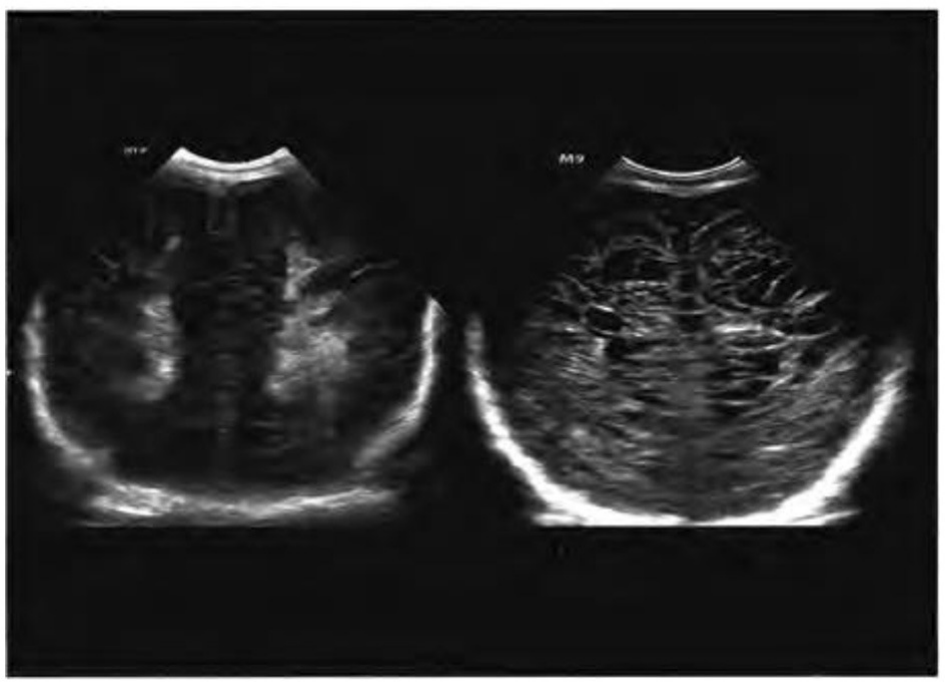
Ultrasonography of periventricular leukomalacia in the same part of the same child (20).
